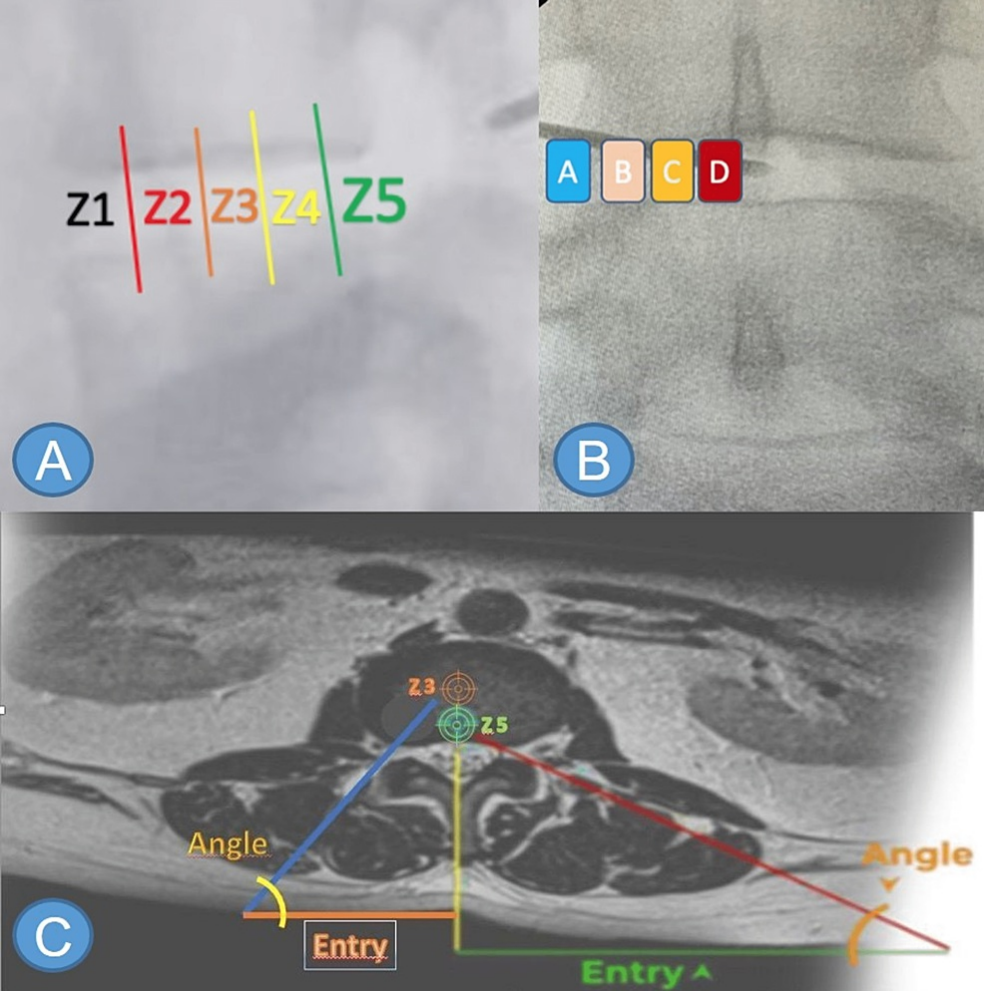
Intervertebral disc zoning, and entry point (A) lateral view radiography showing the lumbar spine. (B) Anteroposterior view radiography showing the lumbar spine. Z1 - "danger zone," close proximity to the abdominal cavity; Z2 - mid anterior; Z3 - center of the disc; Z4 - mid posterior; Z5 - adjacent to the canal; A - extraforaminal; B - foraminal; C - posterolateral; D – central. Zone 1 is adjacent to the abdominal structures and great vessels, Zone 3 is the center of the disc, and Zone 5 is the region close to the canal. (C) projection of the hernia's location (seen on MRI during intraoperative radioscopy). Puncture planning in the transforaminal access (T2 axial cross-section in MRI): Z3: center of the disc - foraminal and extra-foraminal hernias; Z5: adjacent to the canal - central and posterolateral hernias. Source: author
